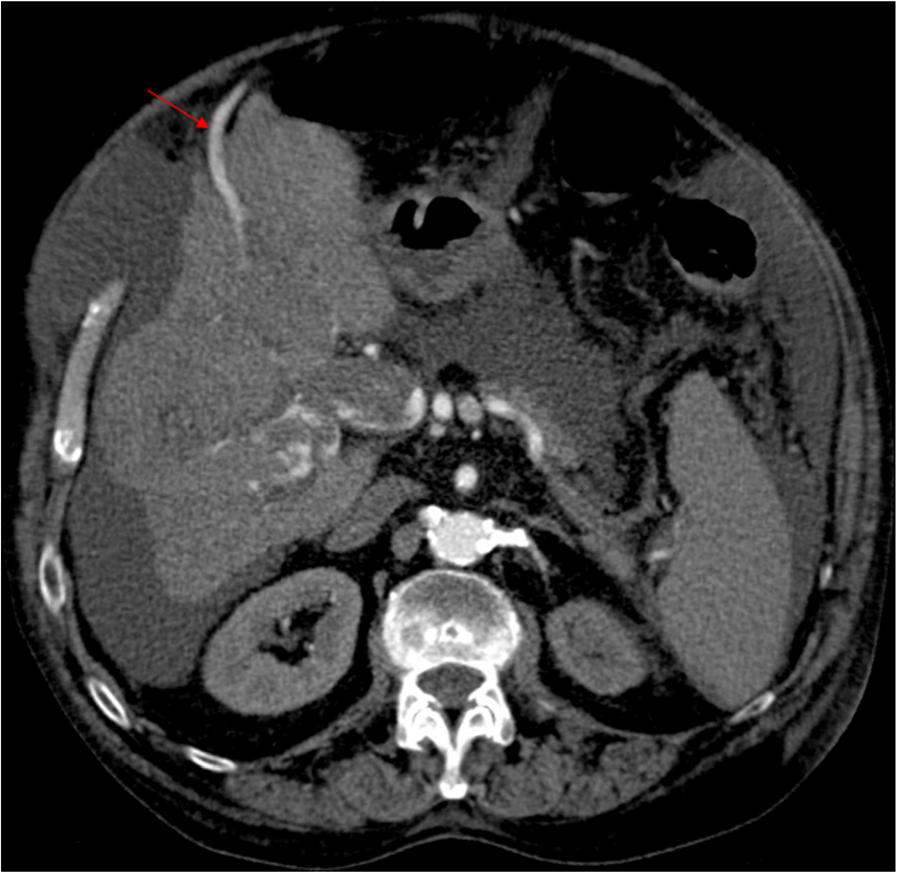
Tumoral PV thrombosis. MPR image from early arterial phase CT shows heterogeneous enhancing PV thrombus, despite absent enhancement of both splenic and SMV (not shown). Note also the presence of cirrhosis (favoring hepatocarcinoma as the cause of the thrombus), ascites, and repermeabilization of the paraumbilical vein (red arrow), signs of PH. The early enhancement of the recanalized paraumbilical vein also suggests the presence of malignant arterioportal fistula
